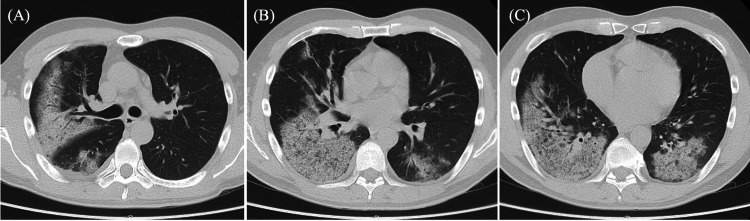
Chest CT imaging on admission. (A)–(C) Axial chest CT scans showing bilateral pulmonary infiltrates with consolidation and ground-glass opacities predominantly in the lower lung.
